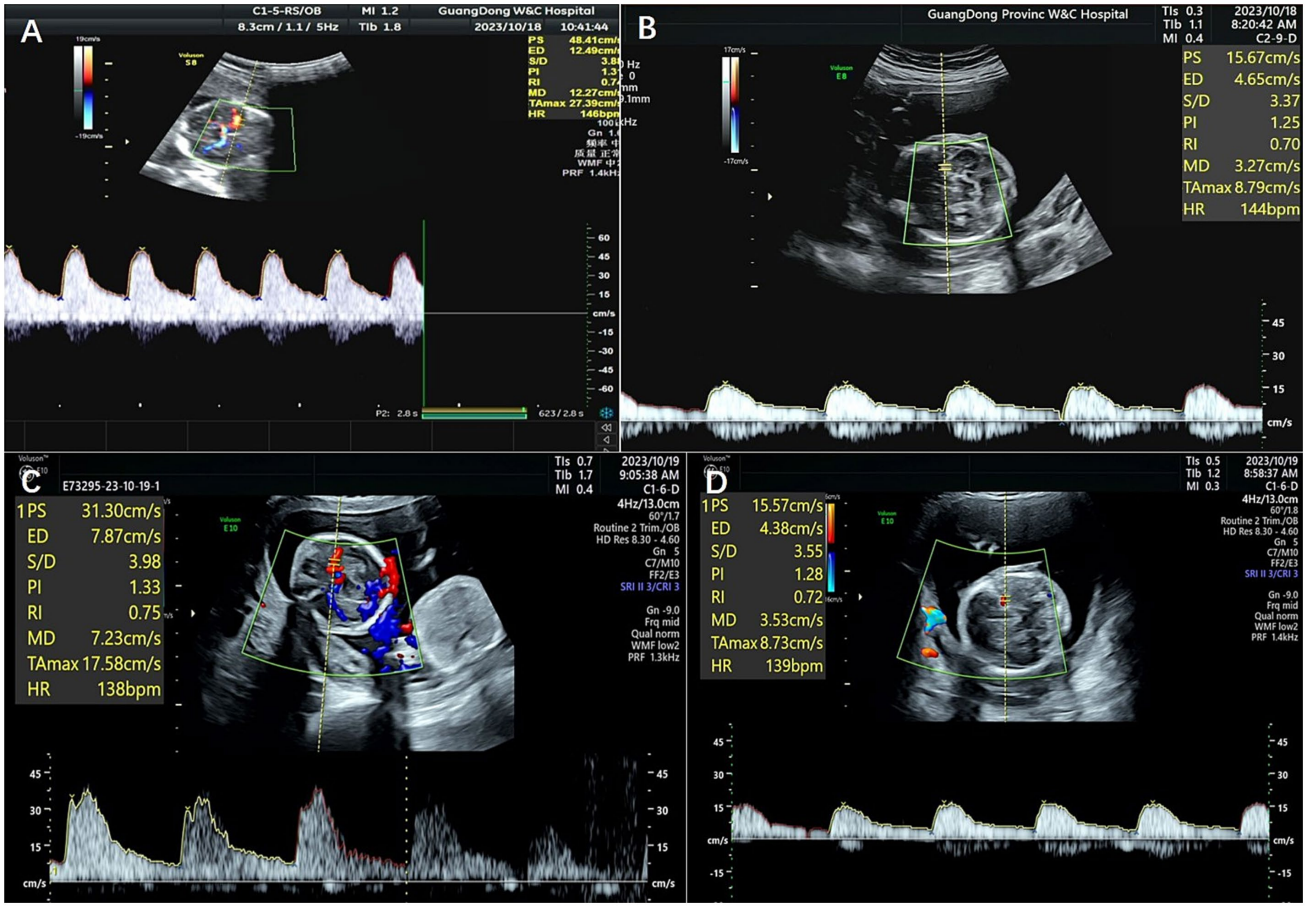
MCA-PSVs on ultrasonography of the fetuses of Patient 2 before and after laser surgery. (A,B) Show MCA-PSVs of the twins before laser surgery, and (C,D) show MCA-PSVs of the twins after surgery.
